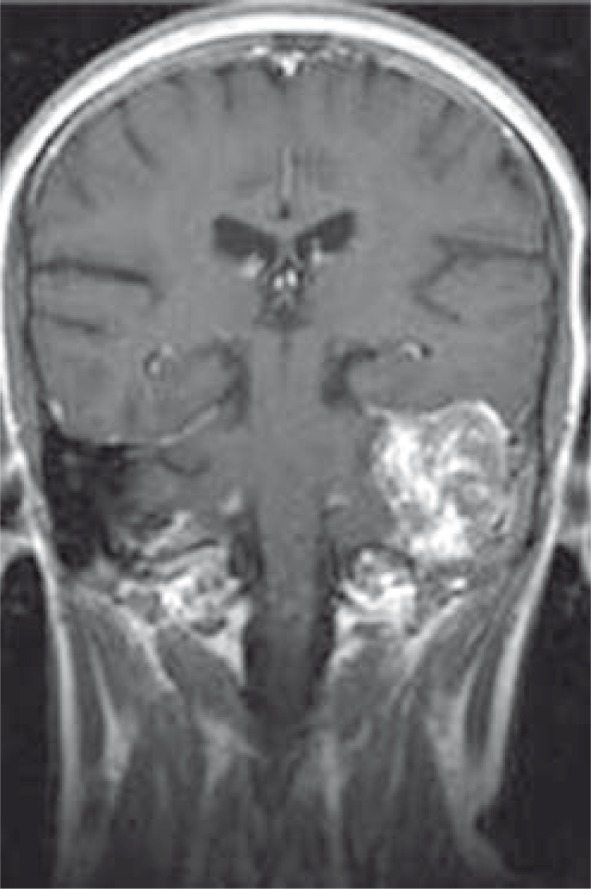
Coronal T1 post-contrast MR image shows endolymphatic sac tumour on the left vestibular aqueduct.
